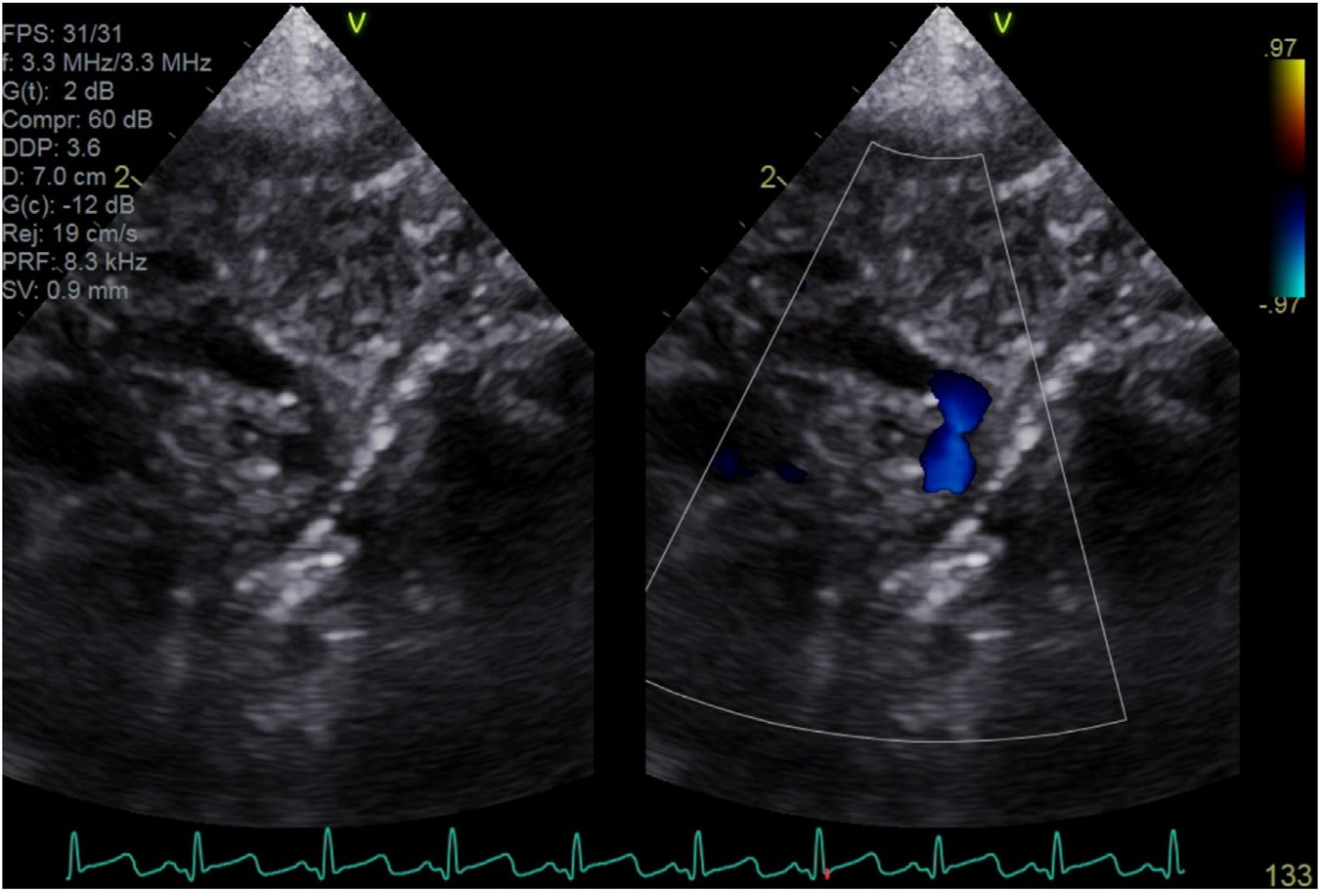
Transthoracic echocardiogram identifying juxtaductal coarctation of the aorta.
